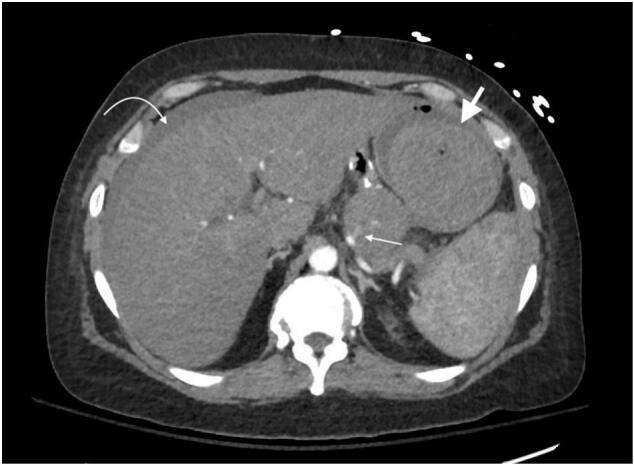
CT angiography. Please observe the active contrast extravasation from the splenic artery (small arrow). There is near complete resolution of the fluid around the gastric remnant (big arrow) and intraperitoneal blood (curved arrow).
